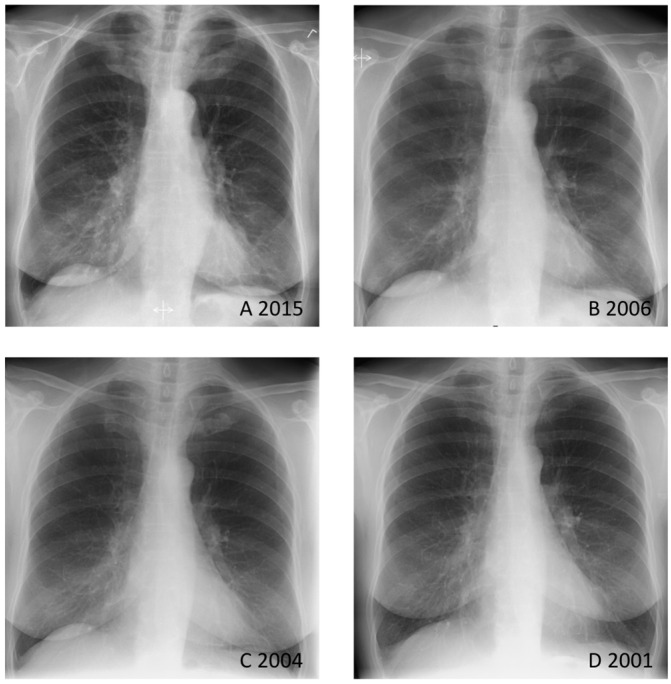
The patient was a healthy 73-year-old woman suffering from intermittent pain in the upper part of the sternum over several years. A chest radiograph from 2015 showed marked bilateral sternocostoclavicular hyperostosis (SCCH) (A); Retrospectively, chest radiographs from 2006 (B) and 2004 (C) revealed that sternocostoclavicular sclerotic changes had already begun—especially when compared with a chest radiograph from 2001 (D). Sternocostoclavicular hyperostosis (SCCH) was described for the first time in Europe in 1975 [1]. Shortly thereafter, in 1977, two new patients presented with the same symptoms of painful swelling of the sternum, clavicles, and upper ribs [2]. In the years to come SCCH was observed in patients in association with various skin lesions, and in 1987 the acronym SAPHO (synovitis, acne, pustulosis, hyperostosis and osteitis) was proposed [3]. Today, SCCH is widely considered to be part of the SAPHO syndrome. SAPHO describes the association between osteoarticular and dermatological lesions, and SCCH is only one of many entities which constitutes SAPHO [4,5,6]. In this patient case, no skin lesions were observed, suggesting SCCH as an isolated entity.
